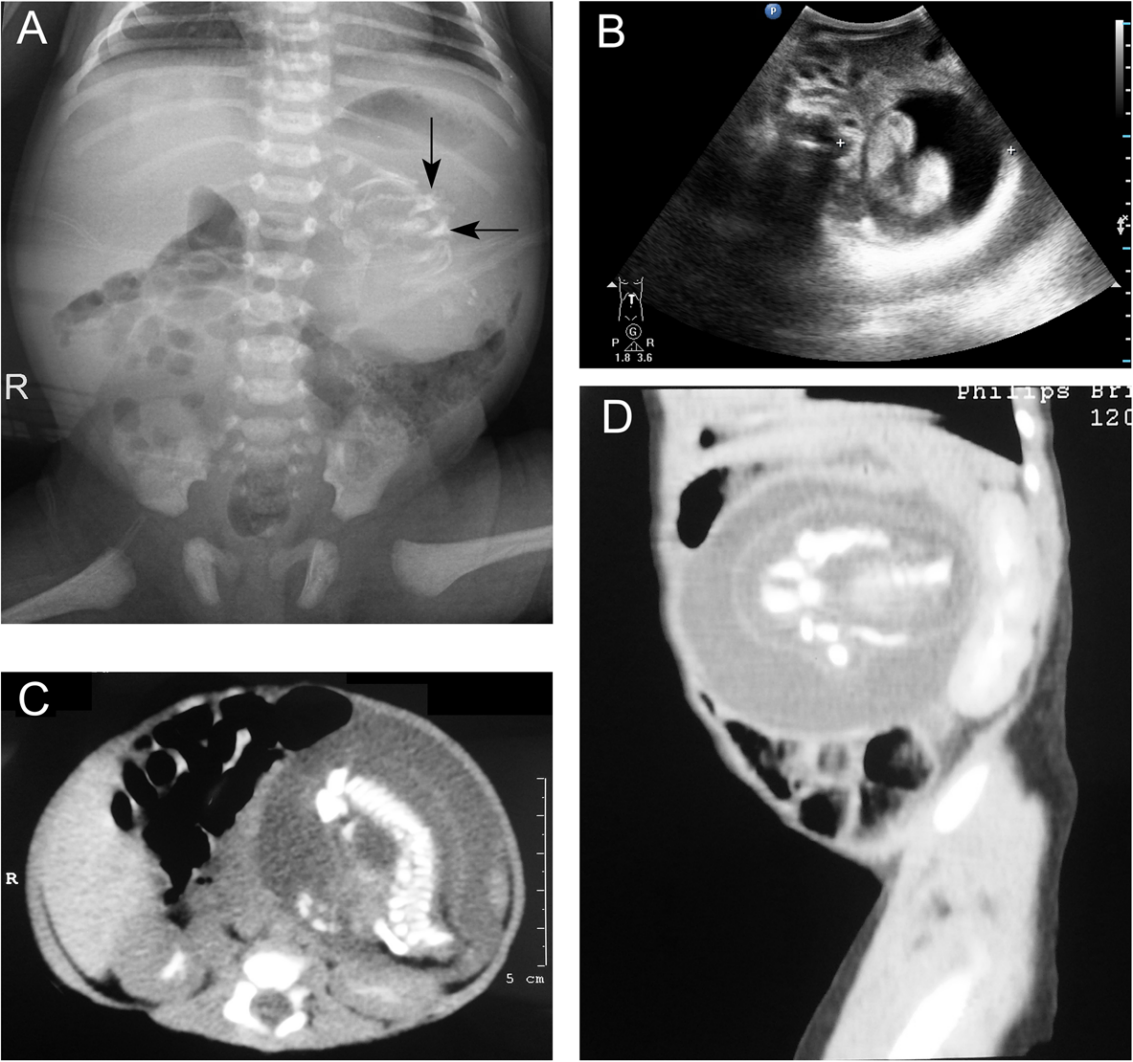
Radiograph, ultrasonography and CT scan of the abdomen showed a large, complex, soft-tissue mass with bony elements. A. Plain film of the abdomen. Calcified mass at the left border of thoracolumbar vertebrae T12, T11, L1 and L2. B. Postnatal ultrasonography of abdomen. The figure shows a retroperitoneal mass in the left upper abdomen. The mass is cystic, with a centrally located fetus. C. Axial CT scan at the L1 level. In front of the left kidney, there is a well-defined mass with 1 cystic formation. There are long and hyperdense opacities corresponding to fetal vertebral column. D. Sagittal CT scan. In front of the left kidney, there is a mass containing fluid and some calcified opacities that corresponding to fetal limbs.
